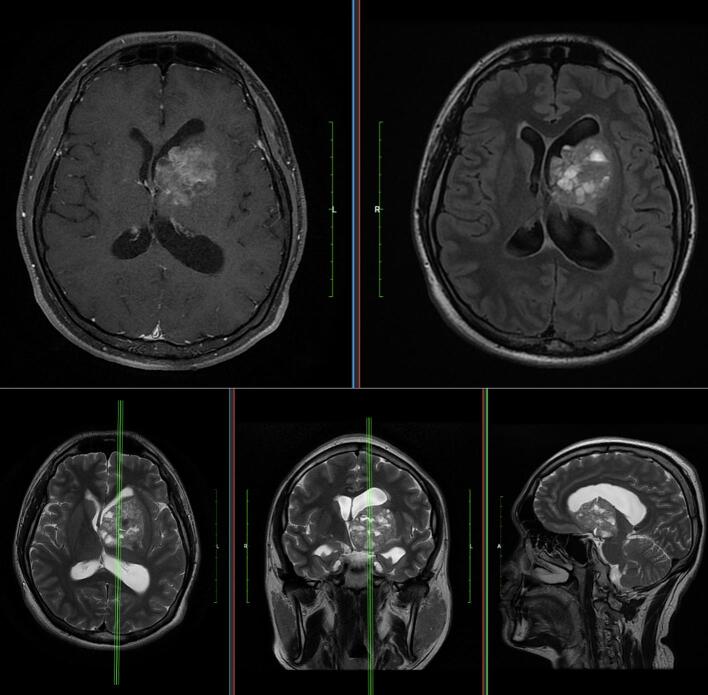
T1, T2, and T2 FLAIR weighted MRI revealing a mixed intensity tumors with multiple haemorrhages in the left basal ganglia region, sizing 4.1 cm × 4.0 cm × 4.2 cm. The mass causing a midline deviation of 10 mm to the contralateral side.
